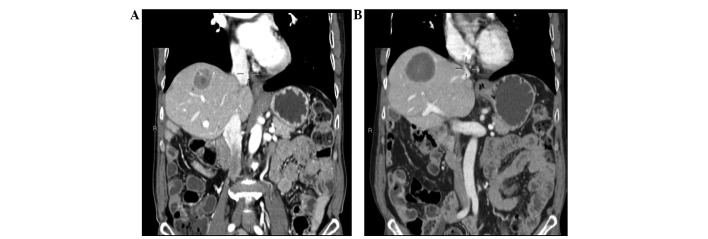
Liver metastasis treated with sunitinib and integrated with treatment using radiofrequency ablation/percutaneous ethanol injection (RFA/PEI). (A) Coronal view of the computed tomography (CT)-scan, following 50 months of sunitinib therapy and 24 months post-surgery, demonstrates a new single liver metastasis in segment VIII. (B) CT scan following treatment with RFA/PEI demonstrating complete destruction of the resistant nodule.
